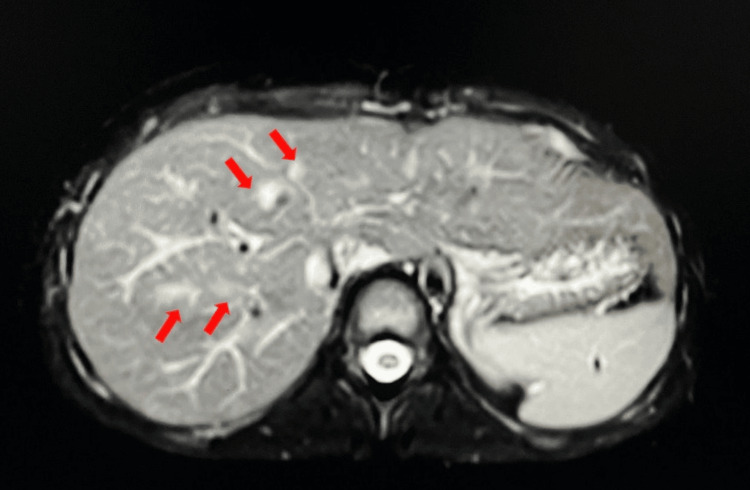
Abdominal magnetic resonance imaging showing multiple hepatic lesions.
